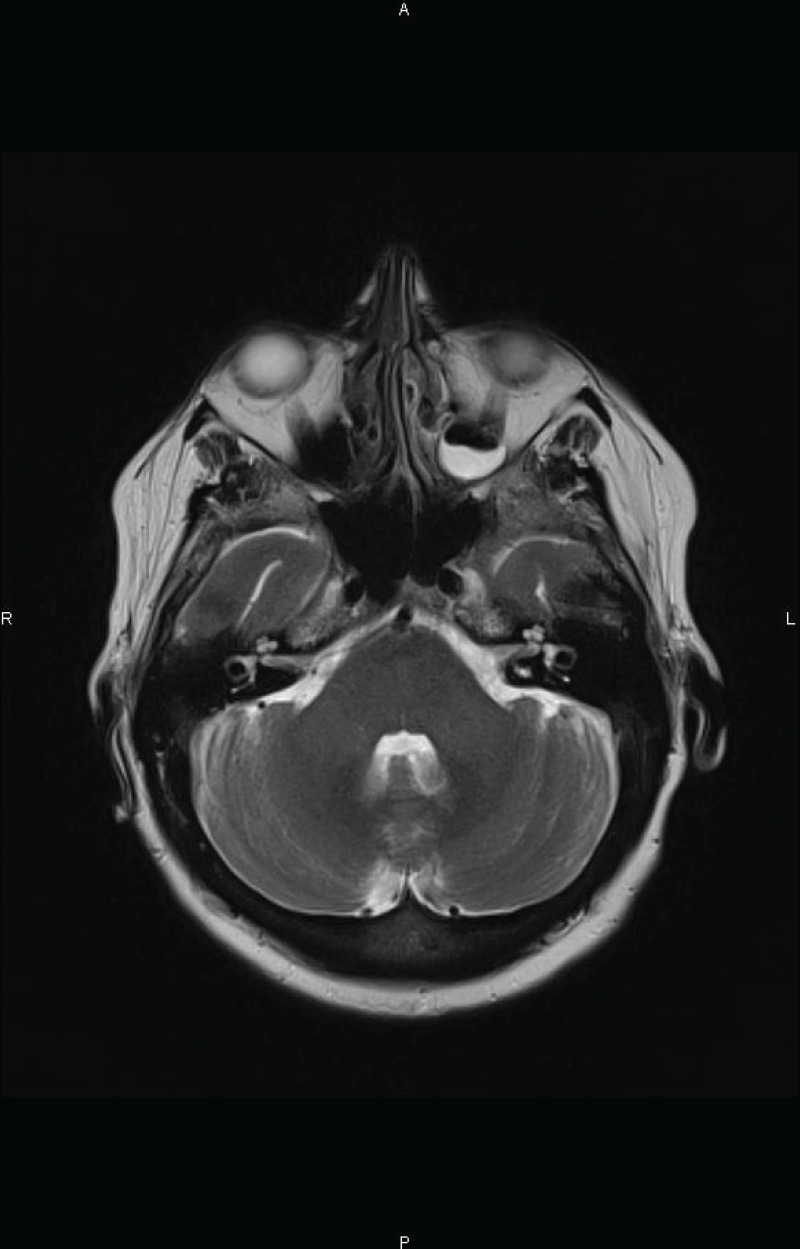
Axial T2 weighted MR image after the conclusion of salvage chemotherapy demonstrating the complete disappearance of the lesion.
